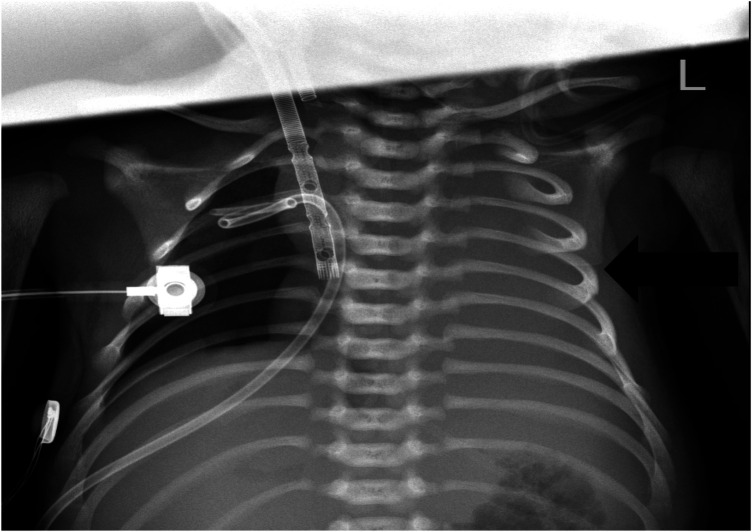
Chest x-ray showing aggravated pneumothorax in the right lung and exudative pleural effusion in the left lung.
